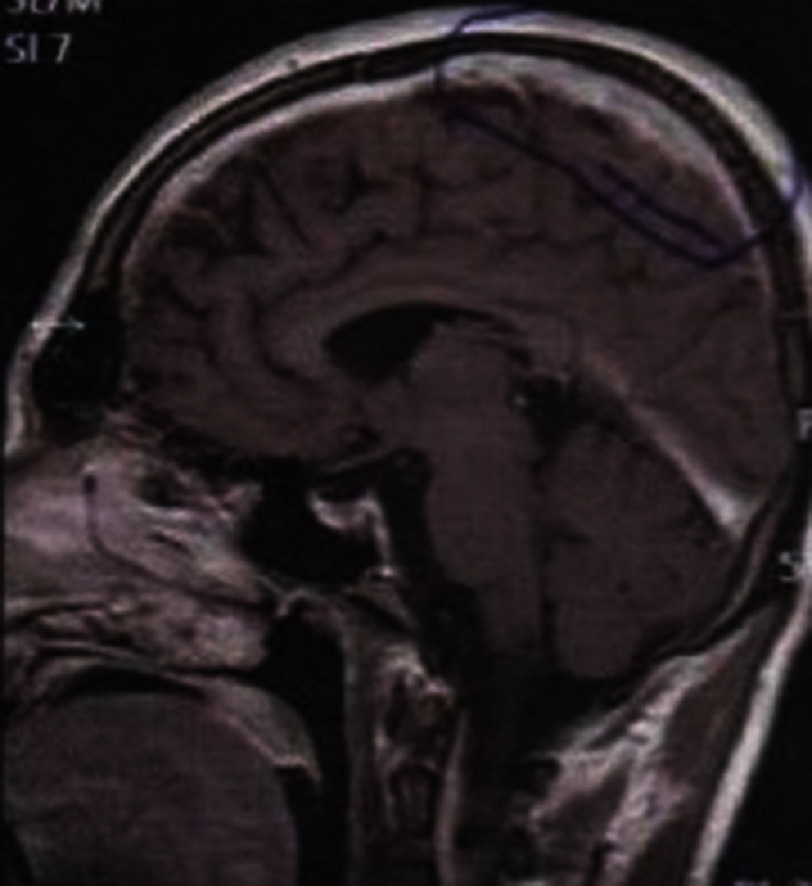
Contrast-enhanced MRI demonstrating thrombosis of the superior sagittal sinus.
